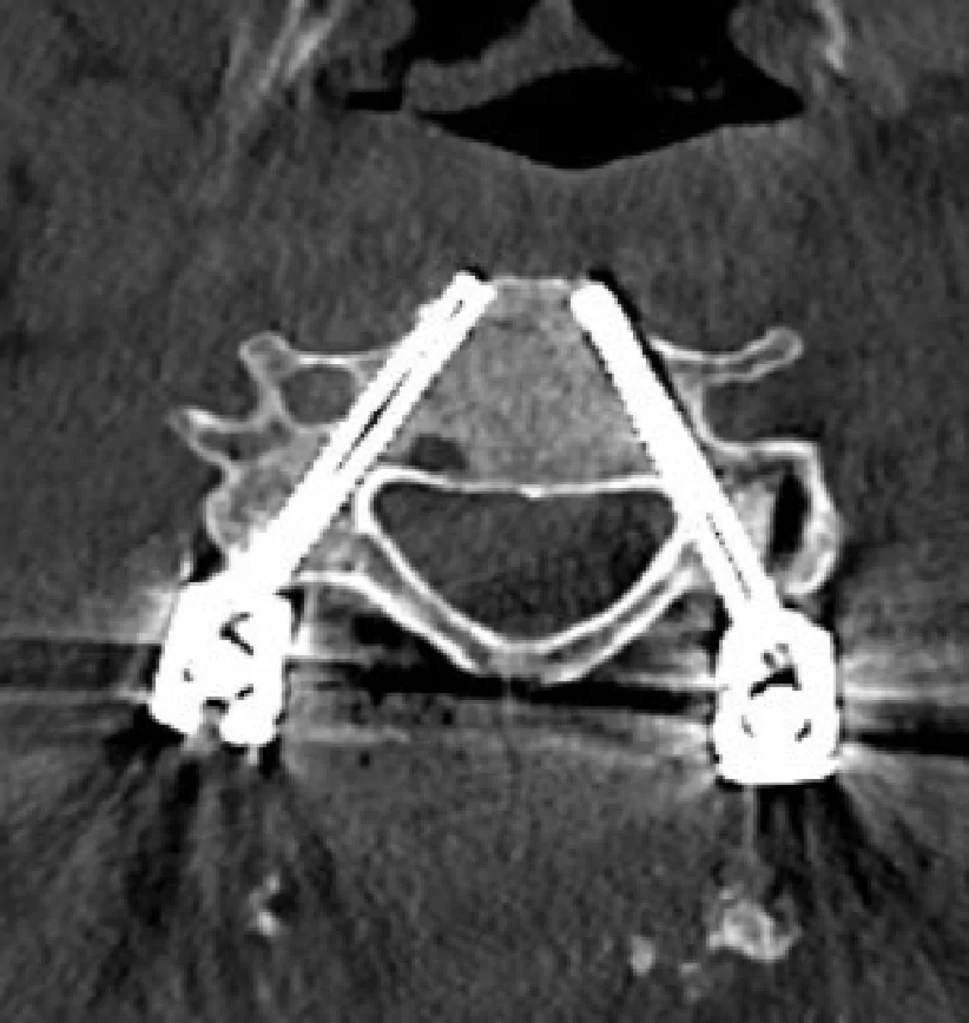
Axial CT pedicle screws C4 (same patient as in figures 3–6 and 8–9). *C*,* cervical spine*
